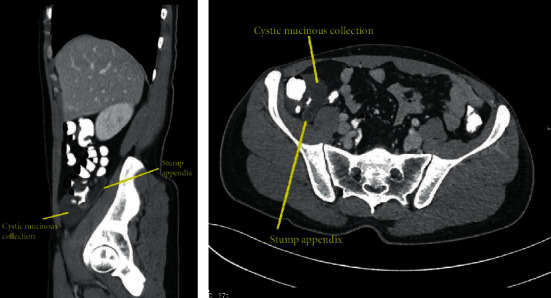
These sagittal and axial cuts from CT scan after appendectomy shows fluid-filled mildly distended appendicular stump with no abnormally thickened wall. An adjacent well-defined fluid collection is seen caudally, showing a density of 20 Hounsfield units.
